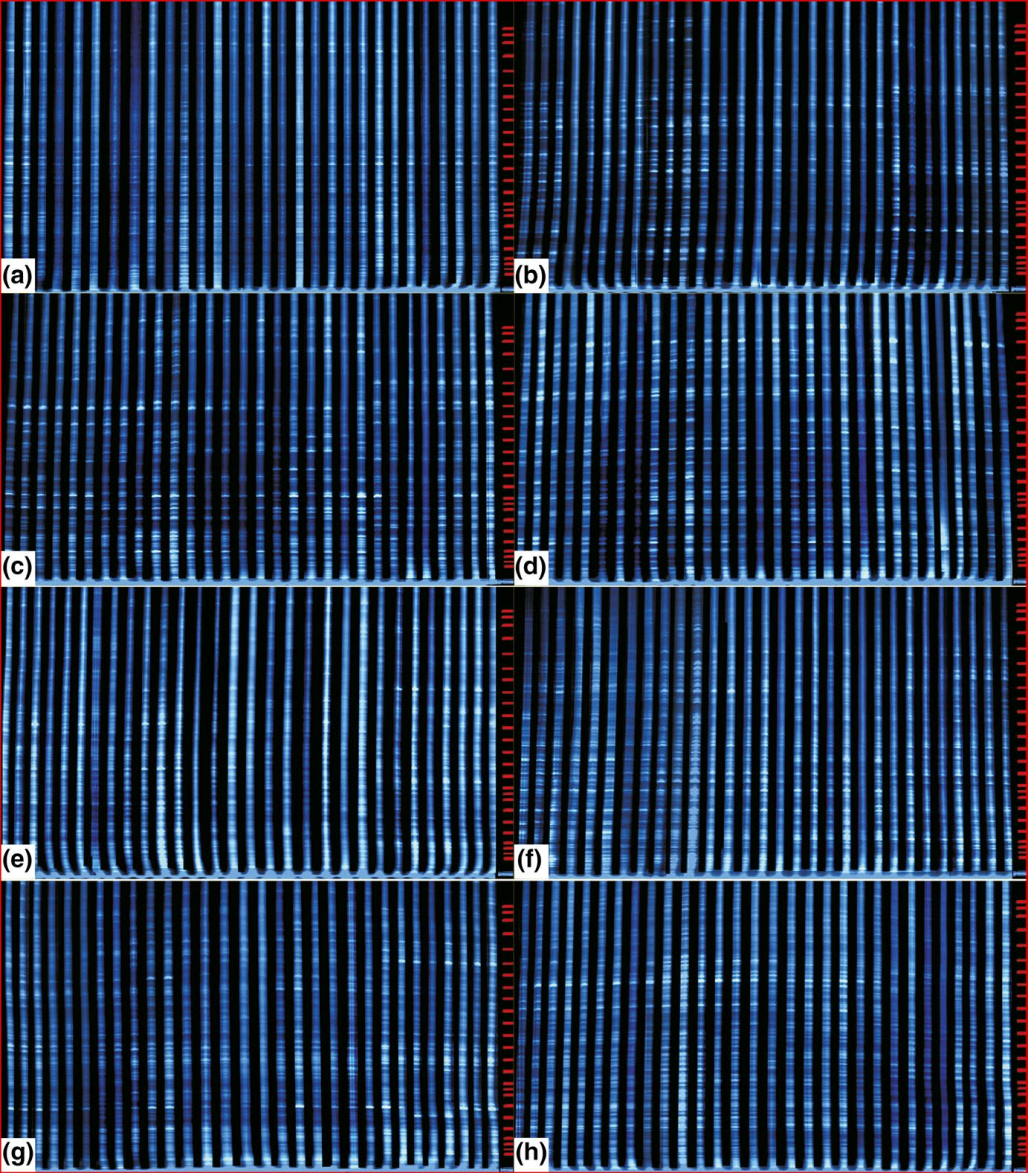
Fluorescently‐labeled AFLPs generated using different primer combinations. (a) P‐GAA/M‐CAA, (b) P‐GAC/M‐CAC, (c) P‐GAC/M‐CTG, (d) P‐GAG/M‐CTA, (e) P‐GAG/M‐CAA, (f) P‐GAG/M‐CAG, (g) P‐GAG/M‐CTG, and (h) P‐GAT/M‐CAG
